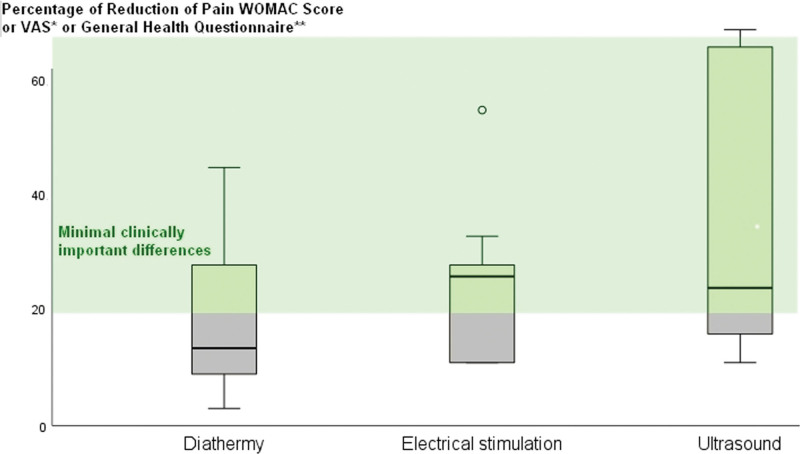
Duration versus percentage of pain reduction in ultrasound studies for knee and hip osteoarthritis. The x-axis represents treatment duration in weeks, while the y-axis signifies the percentage of pain reduction. Studies exceeding the minimum clinical efficiency threshold (set at 20%, based on literature evidence) are enclosed within a highlighted frame for clearer identification and comparison.
